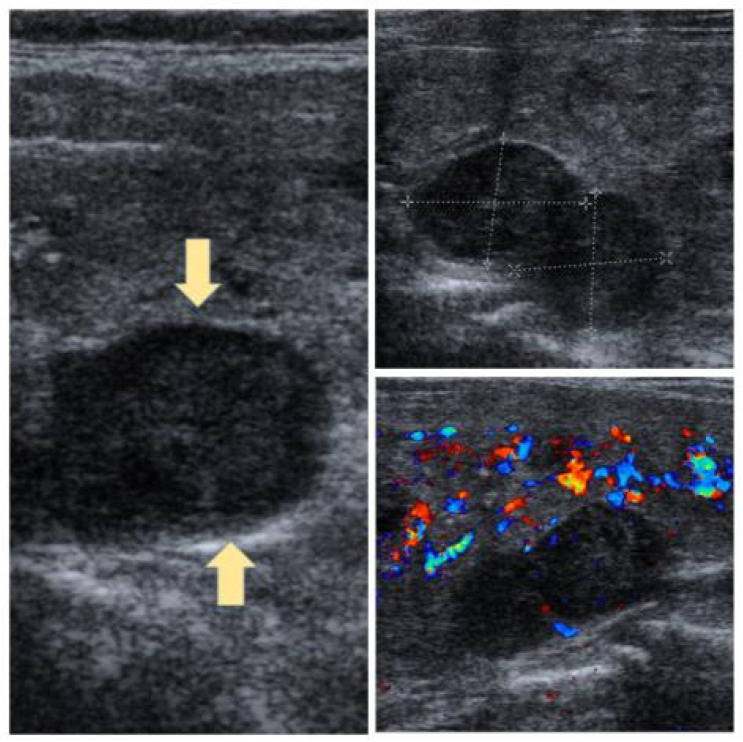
Ultrasound imaging of parathyroid adenoma.
